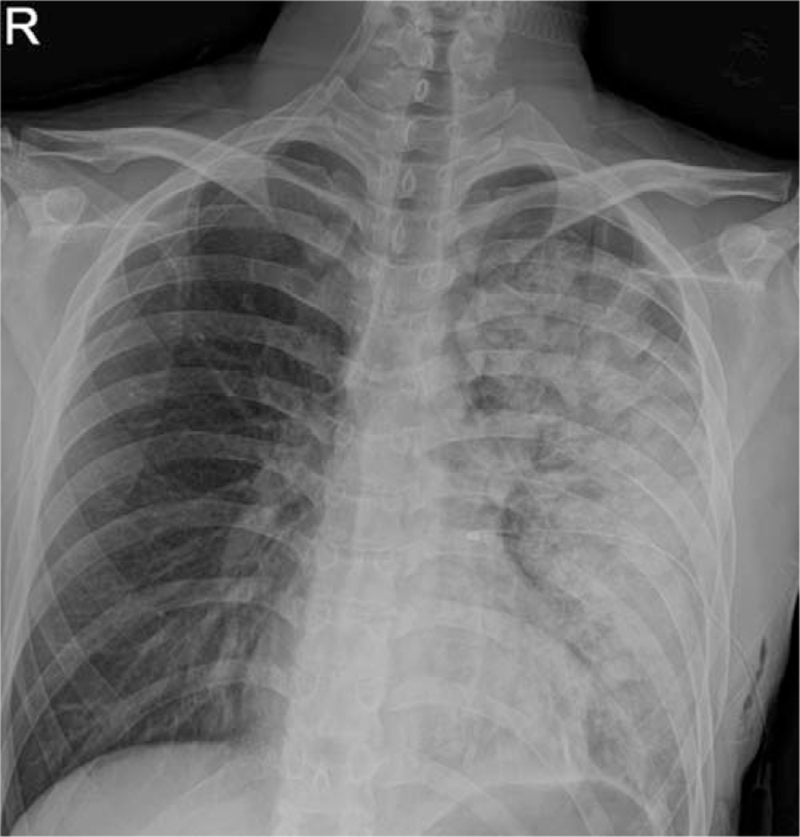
A chest x-ray followed up after the onset of symptoms such as severe breathing difficulties and cough with foamy sputum. A newly developed ill-defined consolidation is observed in the left lung.
